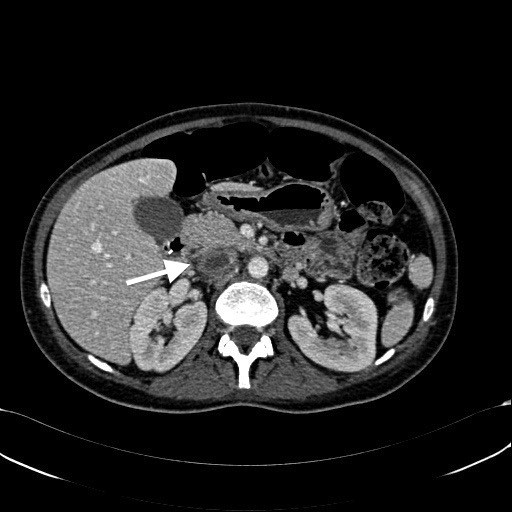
Abdominal Computed Tomography showing complete thrombosis of Inferior Vena Cava.
